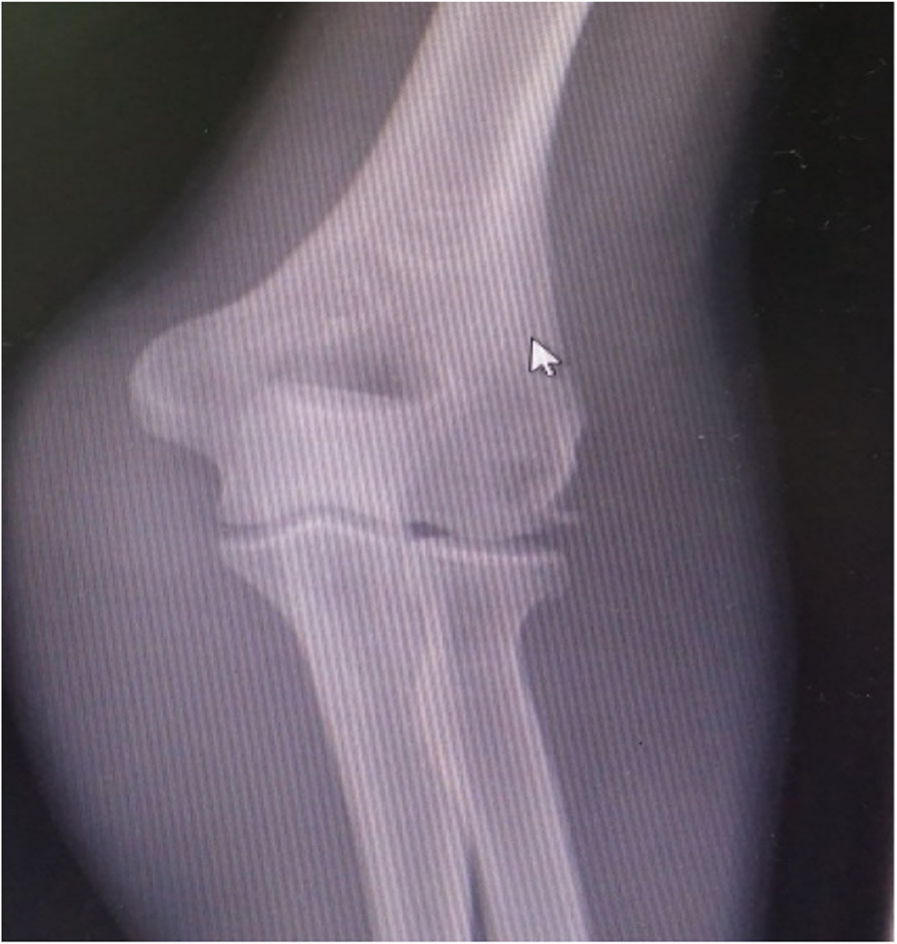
Anteroposterior view radiograph of left elbow. Arrow indicating fracture lateral epicondyle
